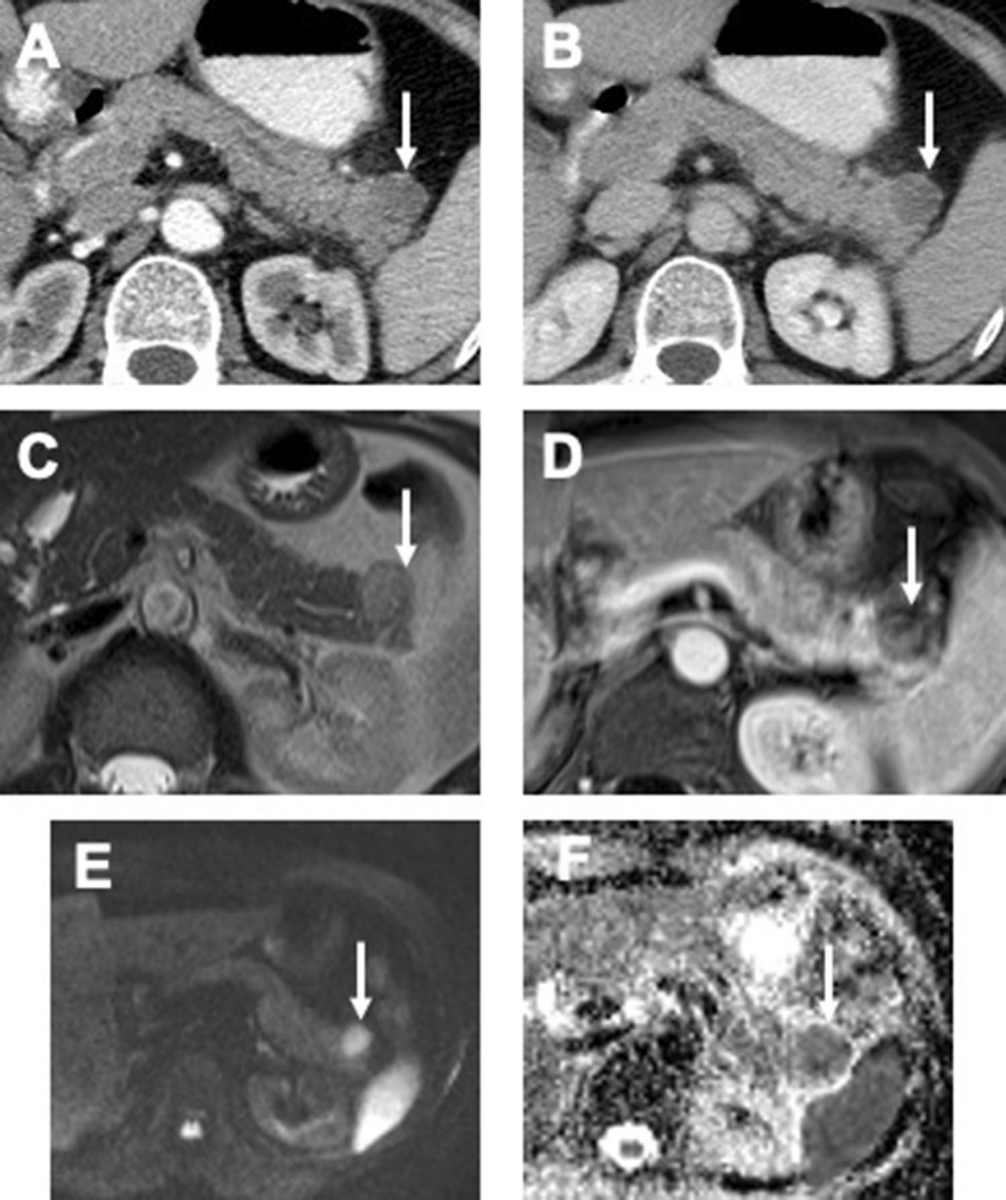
CT and MRI features of ITPN with associated invasive carcinoma. (A,B) Axial CT scans in the arterial phase (A) and portal venous phase (B) demonstrating ITPN lesion in the pancreatic tail (arrows) with well-circumscribed margins and moderate hypointense HU values. (C) Axial T2 weighted image of the same patient, tumor (arrow) doesn’t have classical cystic morphology on T2 but is slightly T2 hyperintense compared to pancreatic parenchyma. (D) Axial T1 weighted image with fat suppression after CM administration, portal venous phase. Tumor (arrow) shows low inhomogeneous contrast enhancement without invasion of adjacent structures. (E,F) Tumor (arrows) shows hyperintensity on axial DWI with b-value 800 s/mm^2^ (E) and hypointensity on ADC map (F), which corresponds the restricted diffusion.
